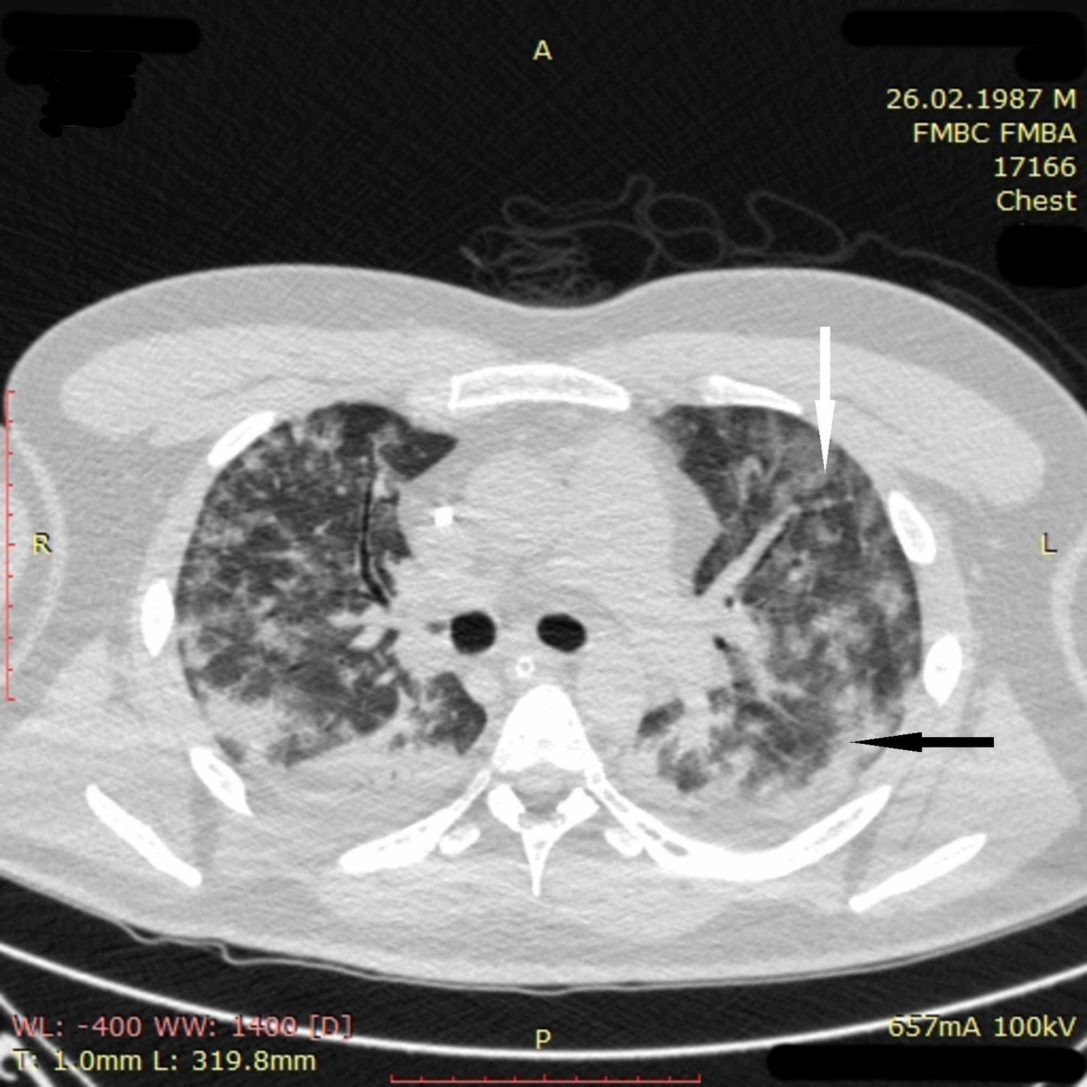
Chest CT imaging of patient 3 on day four CT scan on day four demonstrated the appearance of multiple merging ground-glass opacities (white arrow) and areas of consolidation (black arrow) in all segments of the lungs. Partial atelectasis of both lower lobes was also noted. In general, pathological changes involved more than 75% of pulmonary tissue. CT picture was suggestive of ARDS CT: computed tomography; ARDS: acute respiratory distress syndrome
